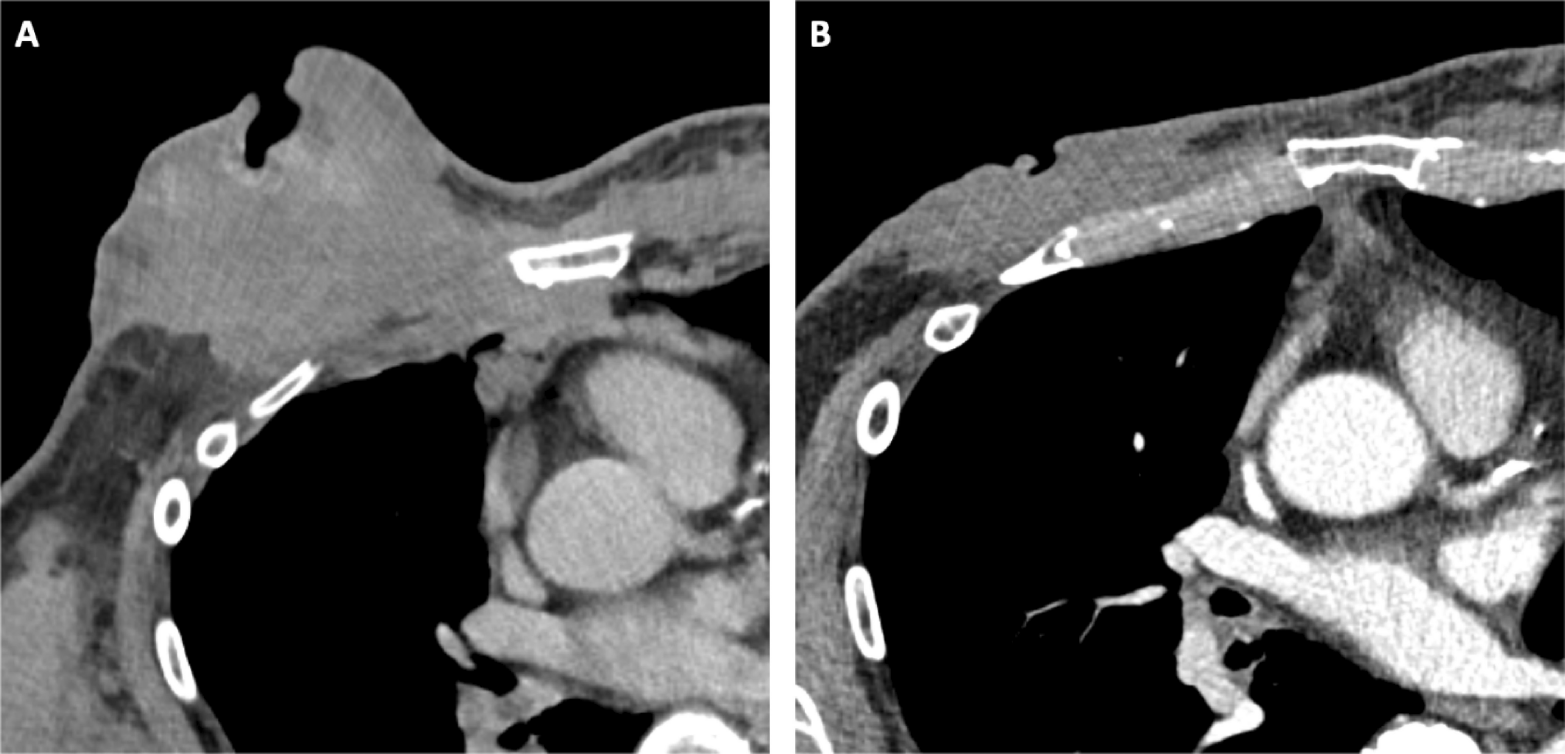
Contrast‐enhanced CT scans of the thorax in the axial soft tissue window before (A) and 6 months after the start of therapy (B). There is a large ulcerating soft tissue tumor in the right mammillary area extending sternally with infiltration of the right pectoralis major muscle, the chest wall and the mediastinum (A). The follow‐up CT showed a clear size reduction of the right mammillary tumor mass as well as chest wall and mediastinal infiltration as a morphological correlate of the clinical response to therapy.
